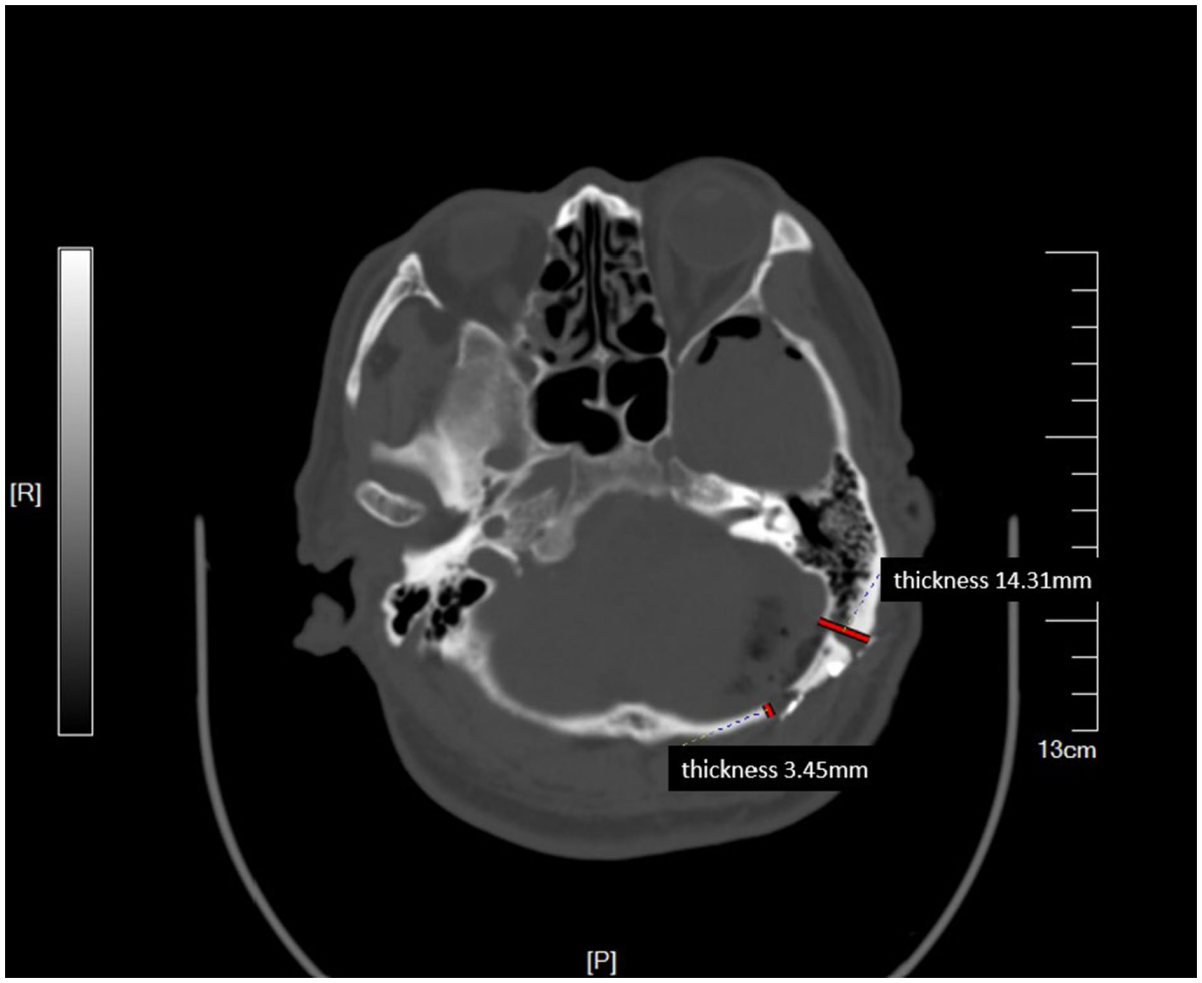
Postoperative CT showing the location of bone flap flush with the sigmoid sinus. The thickness of skull before (45) and behind the bone flap is shown in milimeters.
